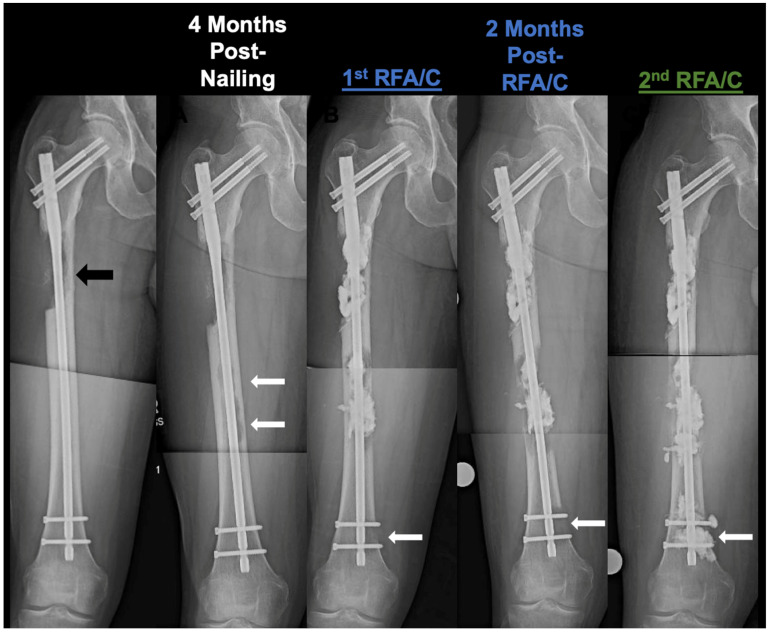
Progressive distal spread of 46M with renal cell carcinoma. Plain X-rays of 46M with metastatic renal cell carcinoma with severe osteolytic lesion progression and cortical destruction of the diaphysis. Received radiation therapy and chemotherapy prior to 1st radiofrequency ablation and cementoplasty. Black arrow depicts initial lesion site immediately post-nailing. Solid white arrows depict the serial progression of the distal osteolytic lesion that may be a result of tumor seeding from intramedullary nailing. A second salvage was required for stabilization of the distal lesion sites. The patient eventually passed away from extensive soft-tissue metastases. RFA/C: radiofrequency ablation and cementoplasty.
